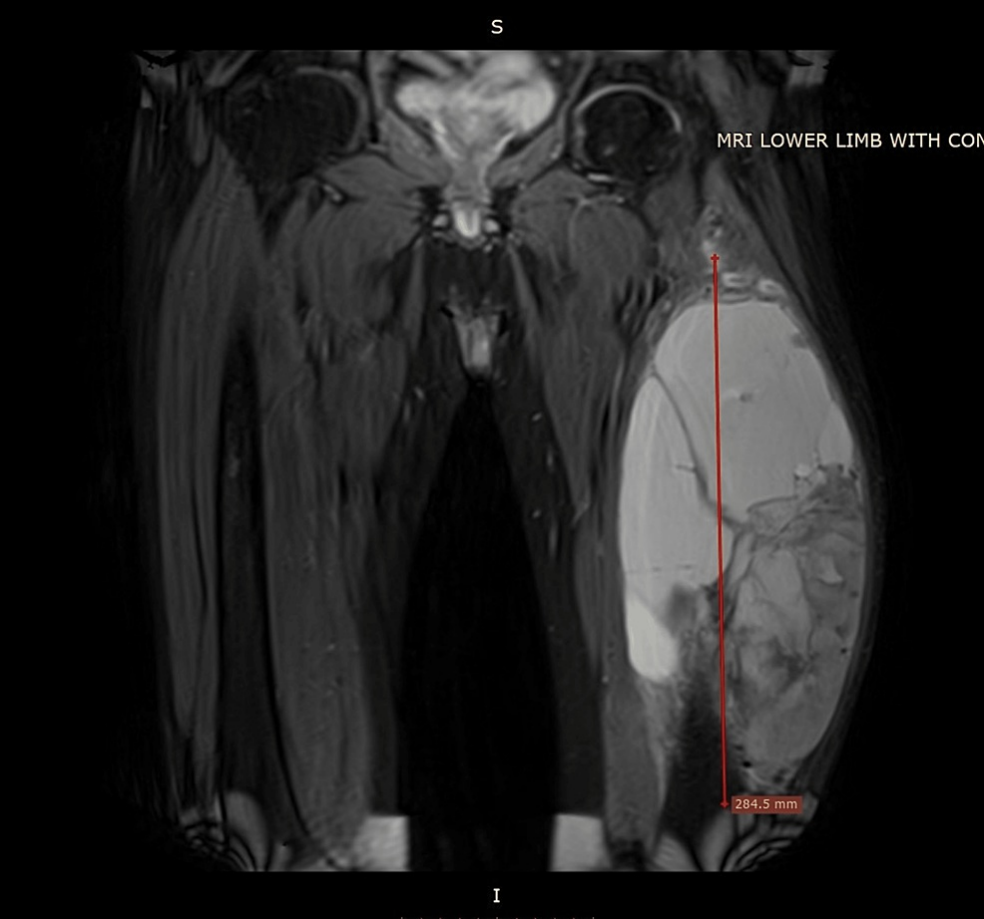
Shows the T1 MRI coronal dimensions of the tumor.
